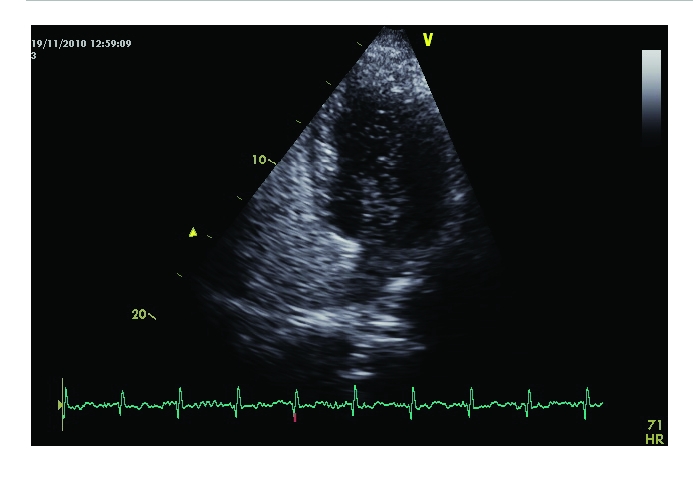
Transthoracic echocardiography, apical 4 chamber view. Immediately after the injection of agitated saline, the contrast is passing from the right atrium to the left atrium, confirming the presence of an interatrial shunt. The contrast is readily seen at the level of the left atrium and passing into the left ventricle.
